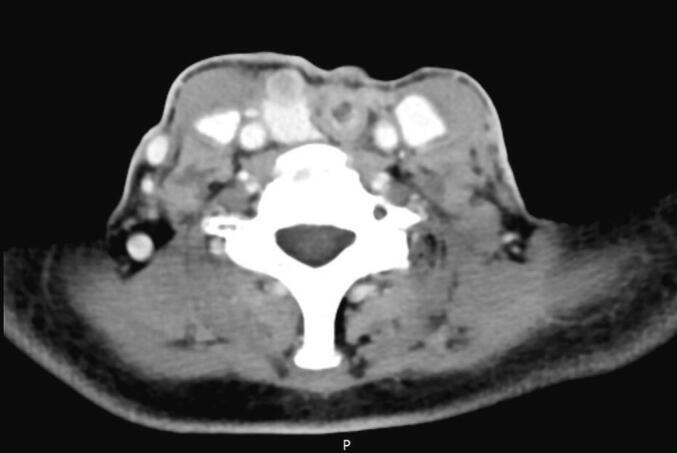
Axial neck CT scan with contrast: round extra-parenchymal lesion with peripheral contrast in the superior pole of the right thyroid lobe.
